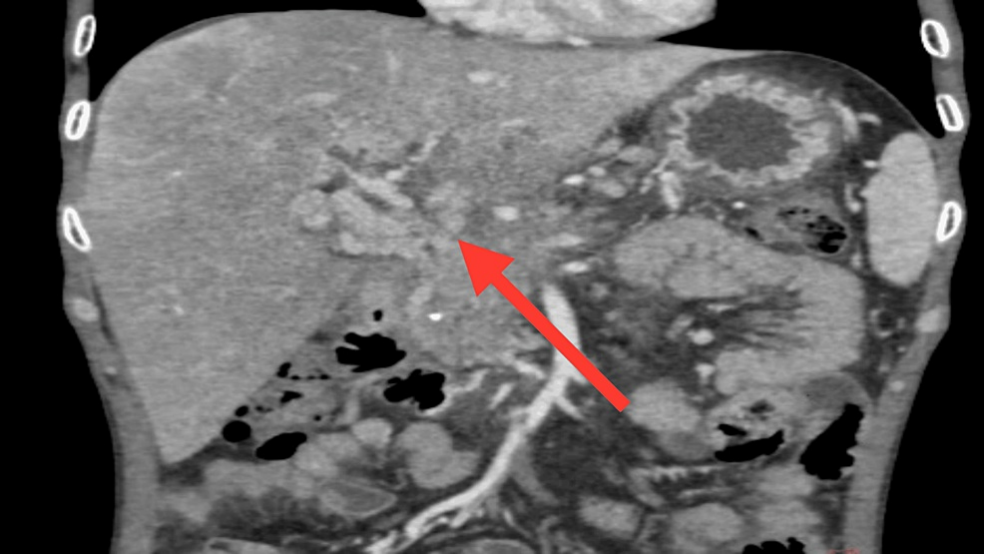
This coronal CECT abdomen section shows coarse echotexture of the liver with portal cavernoma formation (as seen with red arrow) with multiple dilated tortuous collaterals in the peri-pancreatic region, peri-gastric region, para oesophageal region, and at splenic hilum. CECT: contrast-enhanced computed tomography
